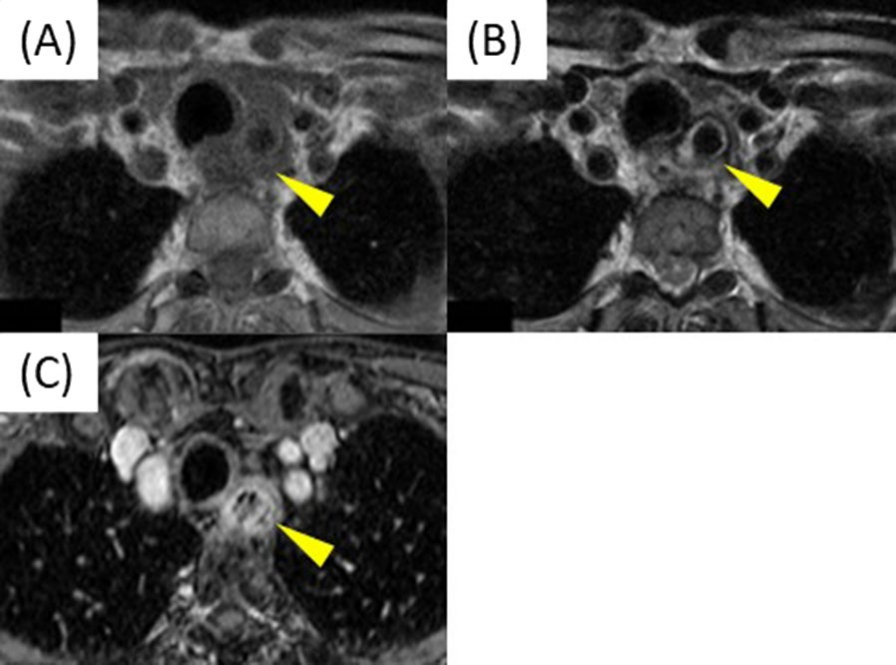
Contrast-enhanced MRI. Plain T1-weighted imaging (A), and plain T2 (B). Contrast-enhanced T1 (C) showed a non-signal line in the mediastinal foreign body
